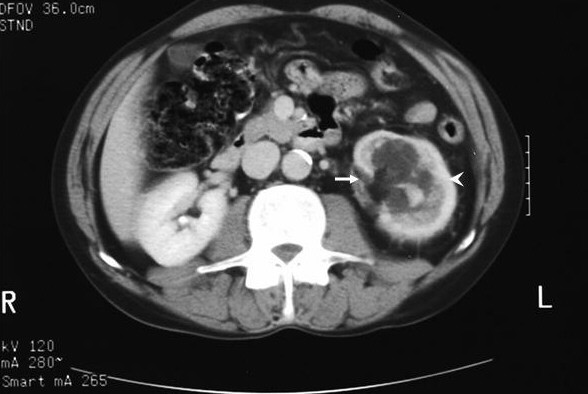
CT scan showing PUJ obstruction (arrow) with ill defined fascial planes around the left kidney (arrow head) that is consistent with inflammation.
